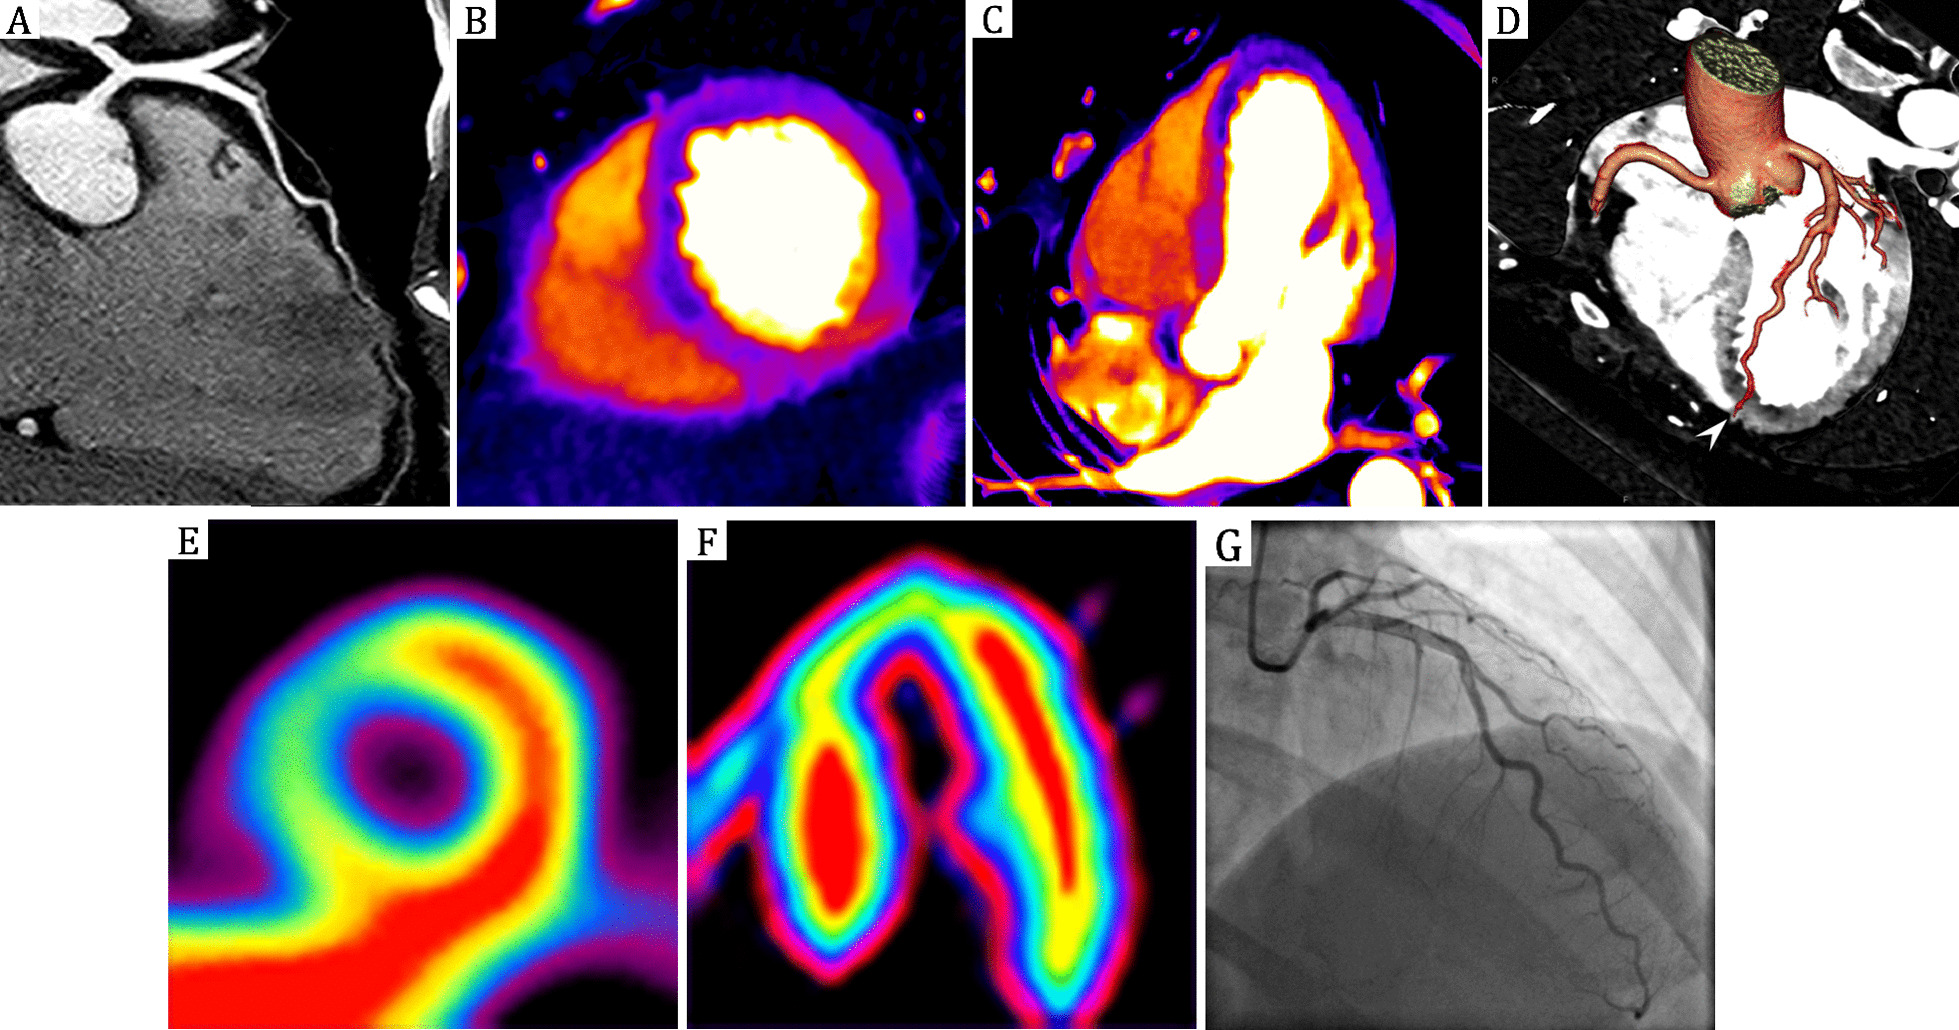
Mismatch between coronary stenosis severity and resting DE-CTP. A Curved multiplanar reconstruction CCTA reveals no coronary artery stenosis along left anterior descending (LAD). But resting DE-CTP analysis multiplanar reformatting demonstrates a rest perfusion defect in short-axis (B) and horizontal long-axis views (C), which confirmed by ^13^N-ammonia PET (E, F). In this 48-year-old female presenting with chest pain, serial troponin was mildly elevated leading to invasive coronary angiography (G), which revealed mild narrowing of the left anterior descending that resolved with intracoronary nitroglycerin, consistent with coronary vasospasm. D Fusion image of three-dimensional CCTA and two-dimensional DE-CTP shows the relationship between the perfusion defects area and corresponding supplying artery (LAD). DE-CTP = dual-energy CT perfusion; CCTA = coronary CT angiography; PET = positron emission tomography; LAD = left anterior artery
